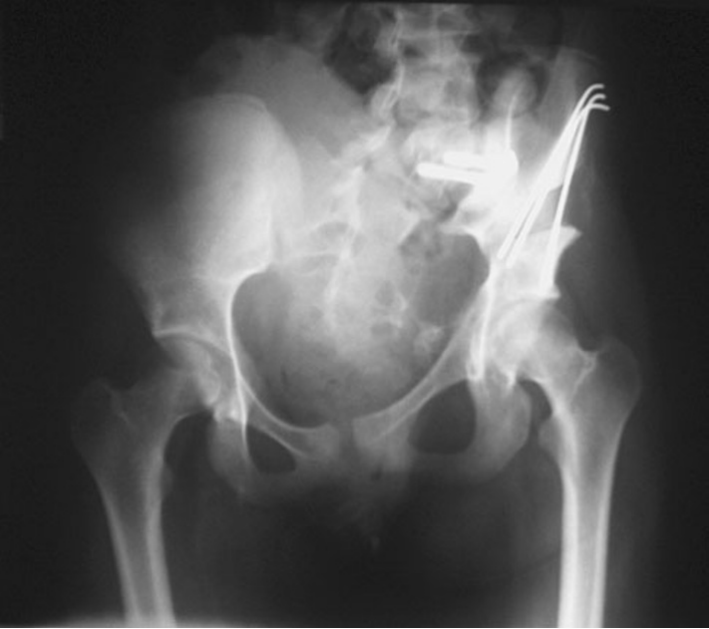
Anterior-posterior pelvis x-ray image shows a transiliac limb lengthening osteotomy using a interposition rectangular bone graft instead of the usual rectangular graft to achieve up to 4 cm of intrapelvic lengthening. It allows correction of certain forms of postural imbalance and pelvic obliquity, as well as allowing an optimal and variable amount of acetabular redirection
